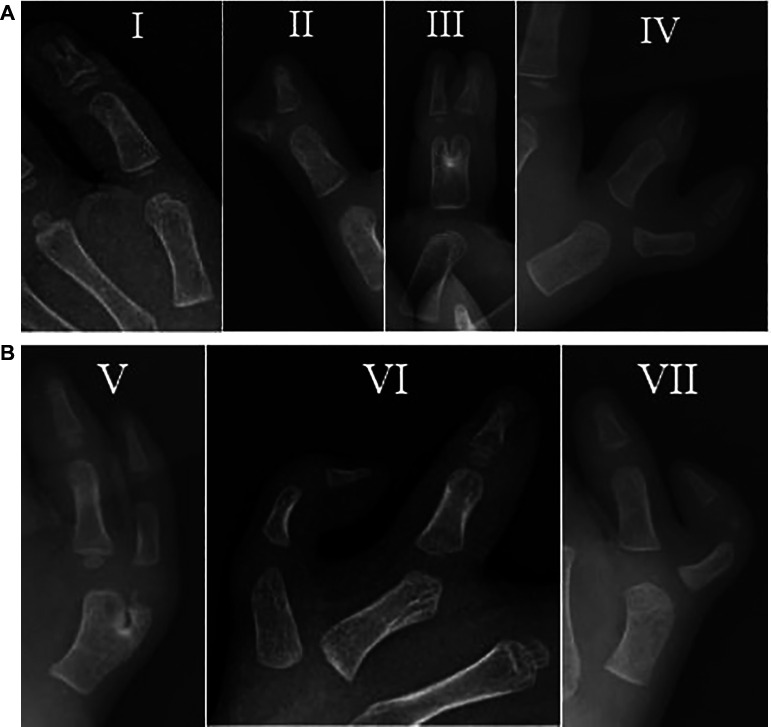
(A,B) Radiograph of the Wassel-Flatt classification: (A) type I, II, III and IV; (B) type V, VI and VII.
